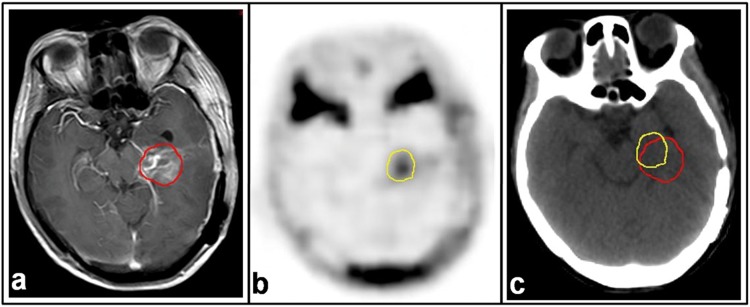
MRI and ^18^F-FLT PET images for a patient with glioblastoma (GBM). Images were taken 21 days post-operatively and 2 days before radiotherapy. a. T1-weighted MRI with contrast enhancement. b. ^18^F-FLT PET images. c. CT image of PET-CT scan. Residual tumor regions defined by T1-MRI (red line) and ^18^F-FLT PET (yellow line) are superimposed on the CT image.
